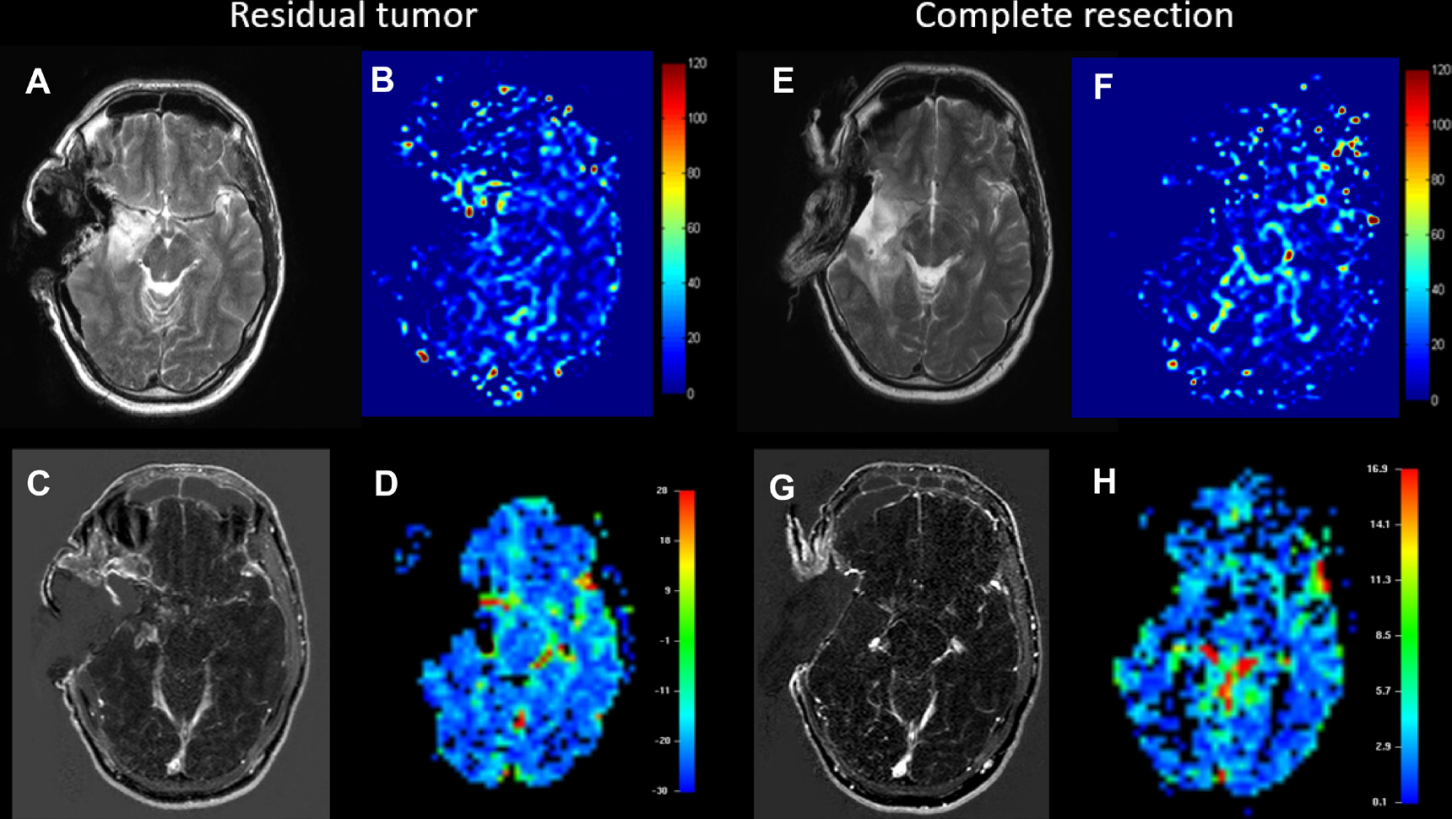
Example of a patient with residual tumor (A–D) and a case of complete resection of the glioblastoma (E–H). A and E show T2-weighted images serving as anatomical reference for the ASL, which is shown in B and F (CBF is displayed in 100 ml/min/100 g tissue). C and G show contrast-enhanced T1-weighted images and finally D and H show negative integral maps of the DSC perfusion (Colormap in arbitrary units). All images were performed using the 1.5T intraoperative scanner.
